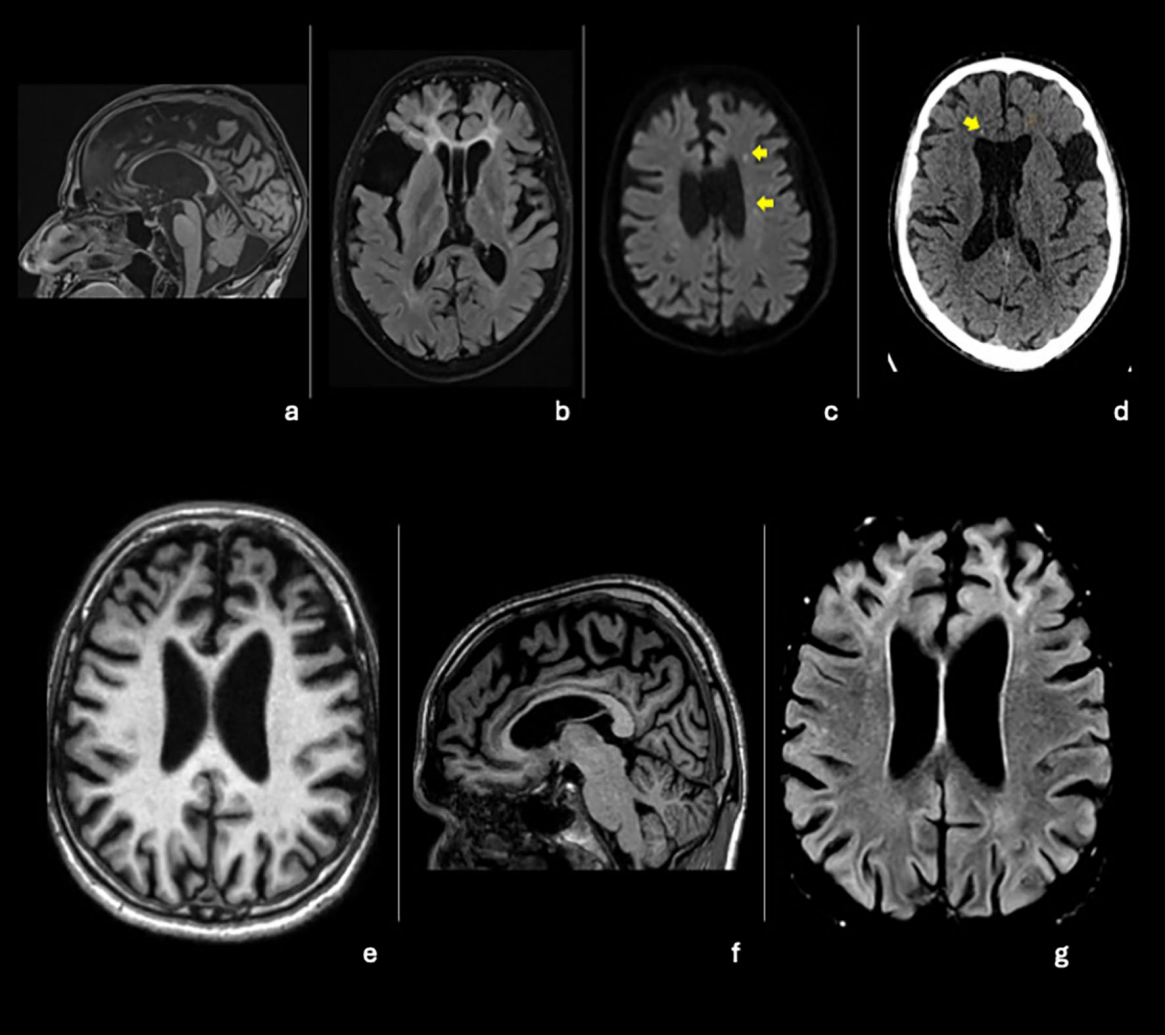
Imaging abnormalities in selected included participants. Brain T2-FLAIR MRI sequence shows corpus callosum atrophy, frontal hyperintensities, cavum vergae, and an arachnoid cyst in patient 2 (a, b). Diffusion-weighted MRI and thin-slice CT (1 mm) (c, d) show the presence of calcifications in patient 2 (yellow arrows). Brain T1-MRI shows frontal atrophy in patient 3 (e); T2-FLAIR MRI sequences reveal thinning of the corpus callosum and frontal hyperintensity in patient 3 (f, g)
